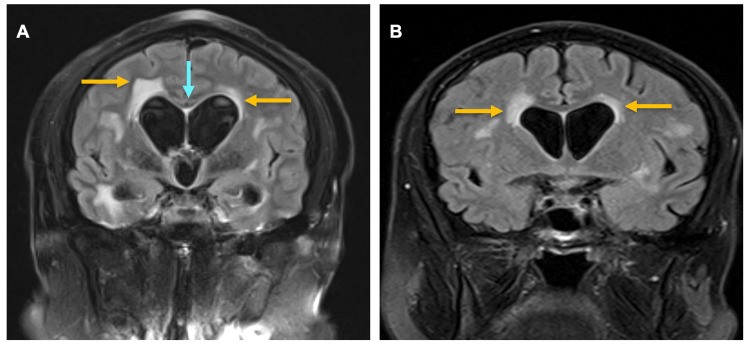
Coronal MRI showing a narrow callosal angle (blue arrow) in Figure 1A and periventricular white matter changes (orange arrow) in Figures 1A and 1B
